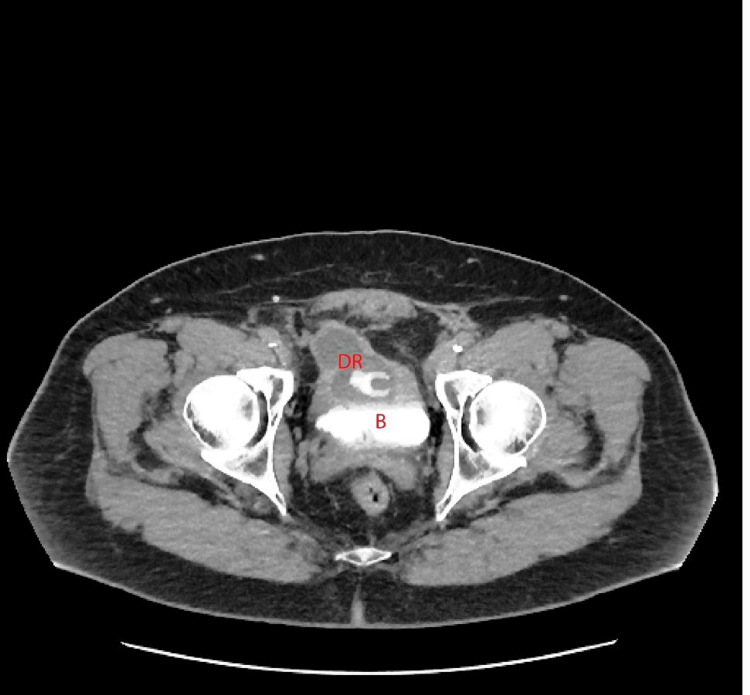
CT urogram transverse section showing DR eroding into the bladder B, bladder; DR, decommissioned reservoir
